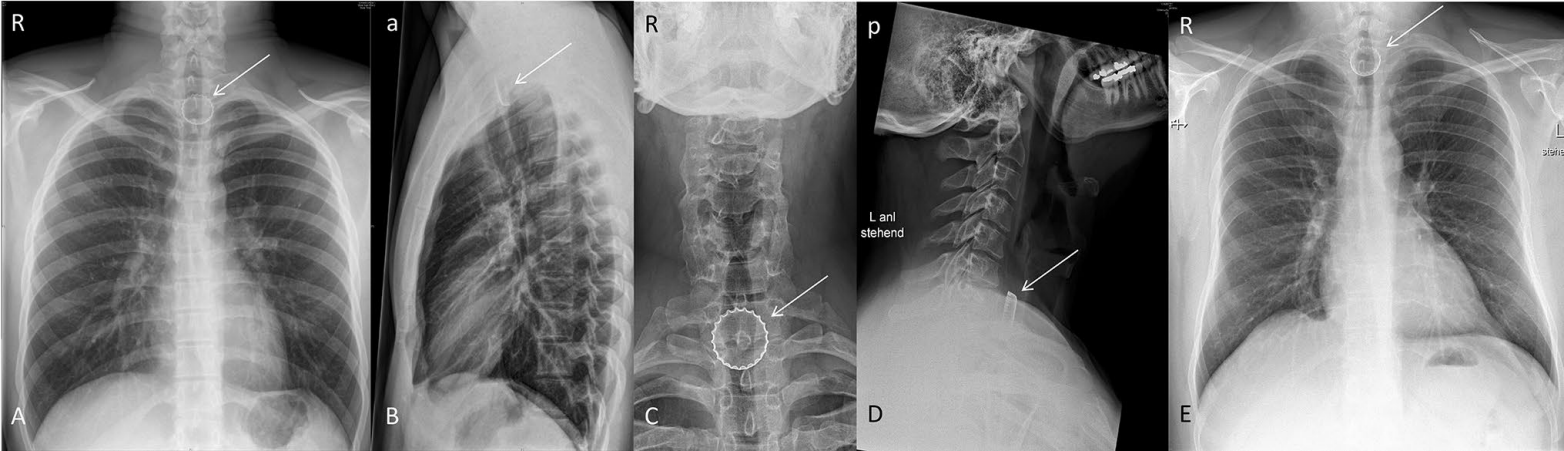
Upper esophageal narrowing. The upper esophageal narrowing lies at the level of near cervical vertebrae VI/VII, about 15 cm measured from the front row of teeth. A, B: Posterior–anterior X-ray (A) and left-sided lateral X-ray of the thorax (B). Bottlecap near thoracic vertebra I/II (arrow). C, D, E: Anterior–posterior (C) and left-sided lateral X-ray of the cervical spine (D). Posterior–anterior X-ray of the thorax (C). Bottlecap near cervical vertebra VII and thoracic vertebra I (arrow). *R* right; *L* Left; *a* anterior; *p* posterior
